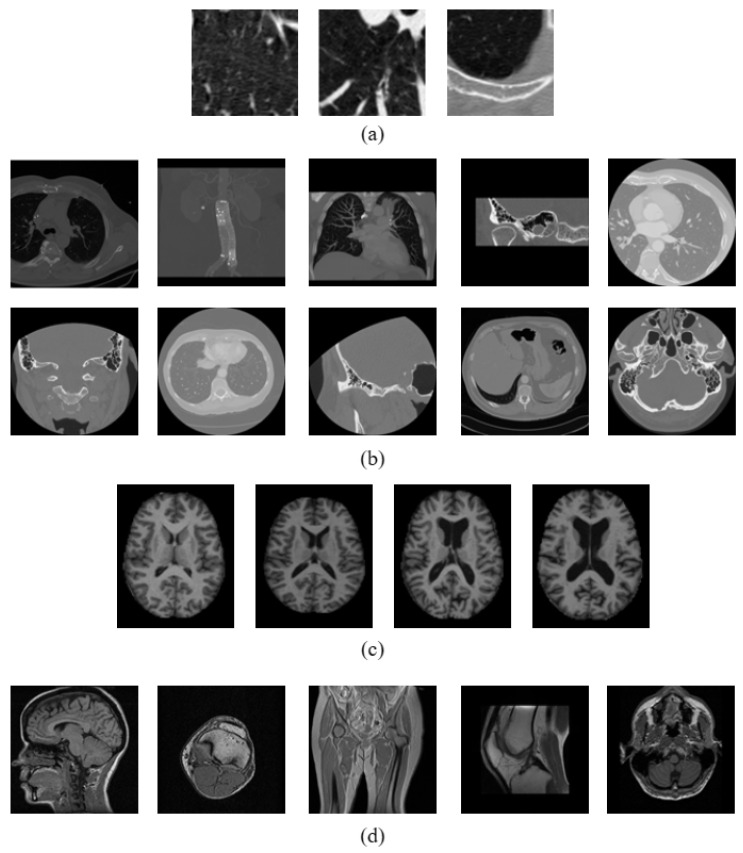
Sample image from each class of (a) Emphysema CT database, (b) NEMA CT database, (c) OASIS MRI database, and (d) NEMA MRI database.
